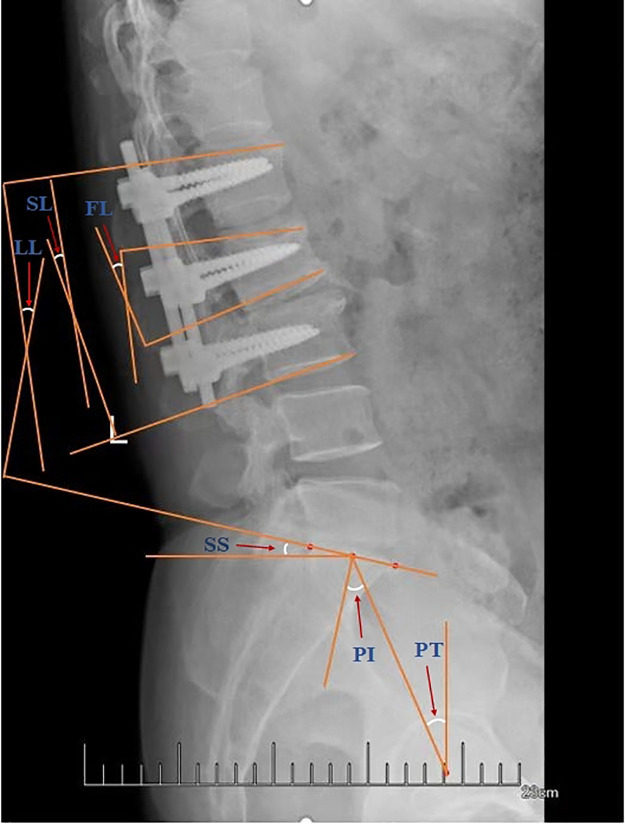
Plain lateral radiograph for measuring radiographic parameters. LL, lumbar lordosis; SL, segmental lordosis; FL, fractured vertebral lordosis; SS, sacral slope; PI, pelvic incidence; PT, pelvic tilt.
